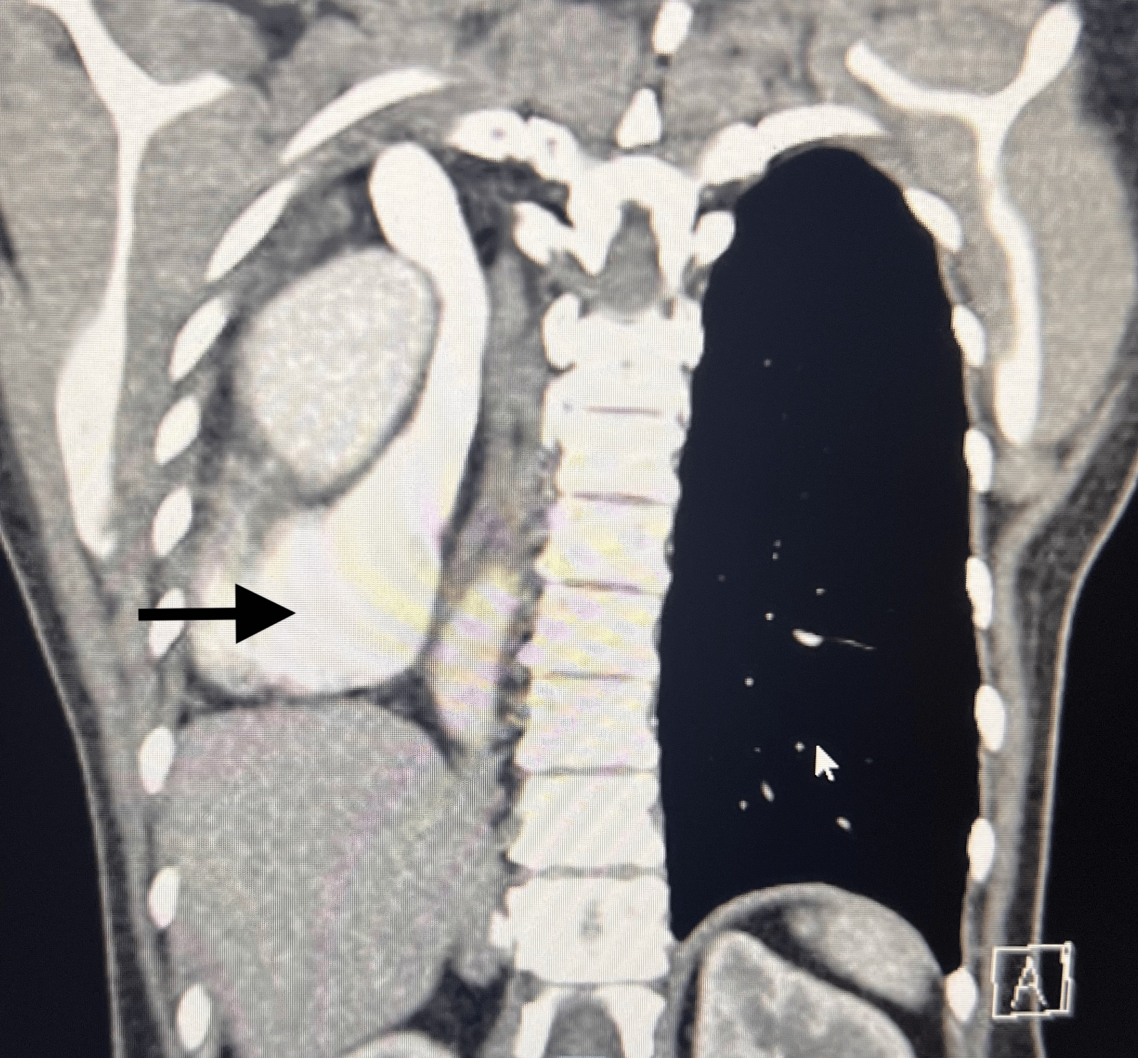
CT chest showing mediastinal structures and the heart lying in the right hemithorax.
